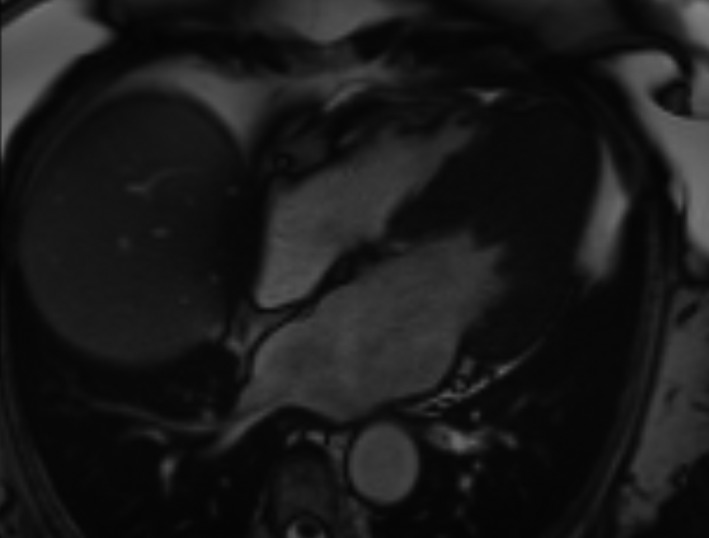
Magnetic resonance imaging of the heart displaying hypertrophic apical and inferolateral septum, appearance consistent with apical hypertrophic cardiomyopathy
